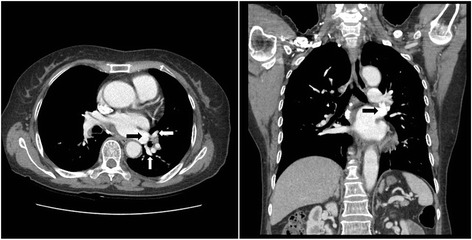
Preoperative chest computed tomography showed that superior and inferior pulmonary veins (*white arrow*) had formed a extrapericardial common trunk (*black arrow*)
